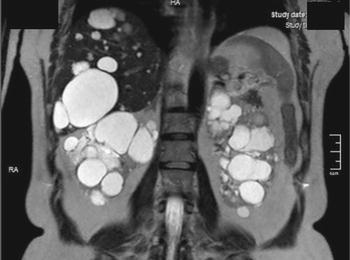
Polycystic kidneys in magnetic resonance imaging
